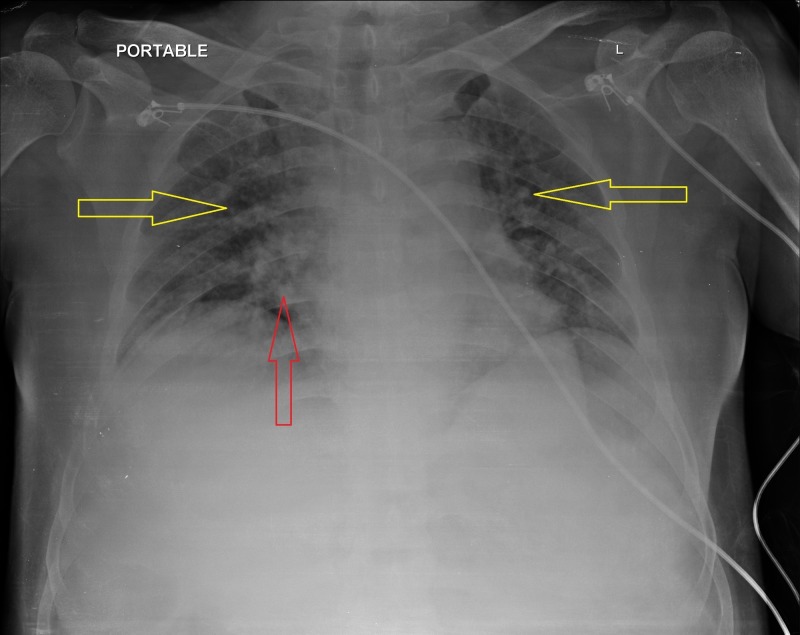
Chest X-ray Red arrow: Hilar and perihilar interstitial prominences. Yellow arrows: Bilateral nodular opacities.
